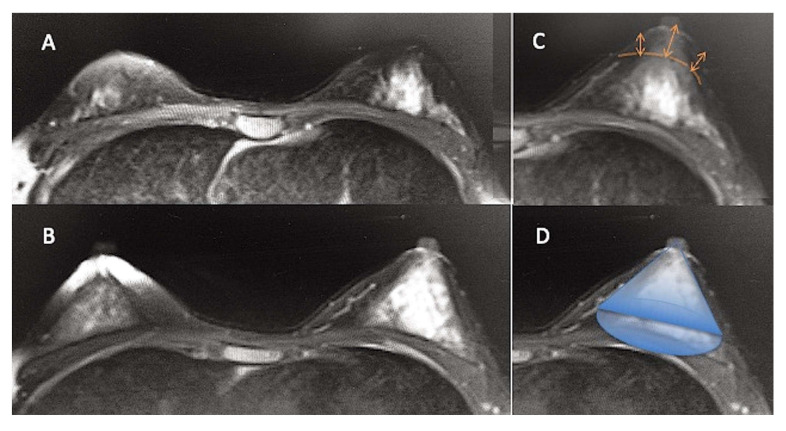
Magnetic resonance imaging (MRI) of a patient treated with AFG. (A) The pre-operative situation in a patient affected by bilateral breast hypoplasia. (B) The post-operative situation at T5 (6 months) after one AFG treatment with an evident improvement of bilateral breast volume. (C) Overlapping of pre-and post-op MRI images with the volume increase measurement detail of 29.5 mm (arrows). (D) Overlapping of a geometric cone on augmented breast to note the similar aspect of the breast to a cone.
